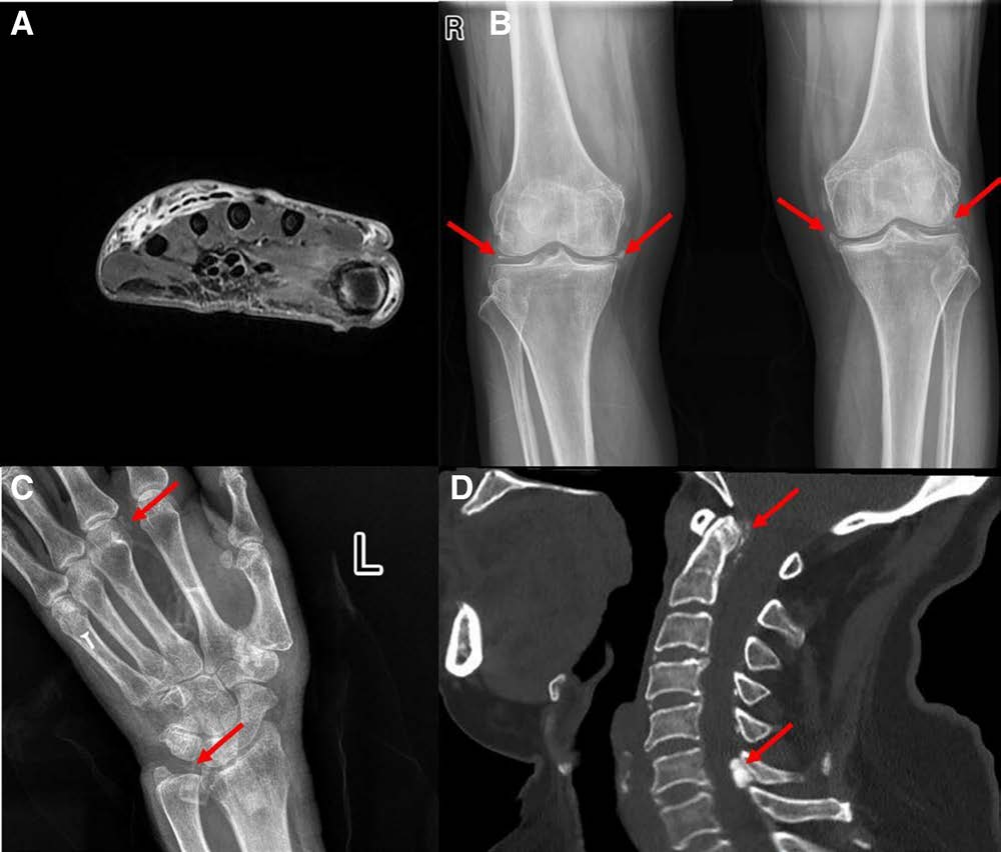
(A) MRI of the left hand showing diffuse soft tissue swelling. (B) X-ray of the knees showed bilateral osteoarthritis with linear calcification of the menisci in both knees. (C) Left wrist X-ray showed calcification of the triangular cartilage and calcification around the third metacarpophalangeal joint. (D) CT images of the cervical spine showed calcification of both the transverse ligament and the ligamentum flavum. CT = computed tomography, MRI = magnetic resonance imaging.
